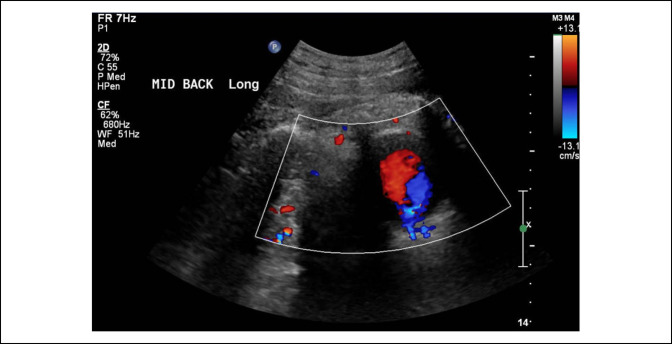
Radiograph showing color Doppler US of the back showing a bidirectional swirling flow consistent with the pseudoaneurysm.
